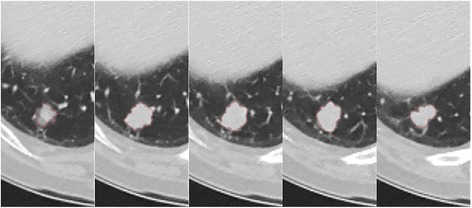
ROI placement in five serial CT slices of a patient with EGFR (+) lung adenocarcinoma in the right lower lobe
